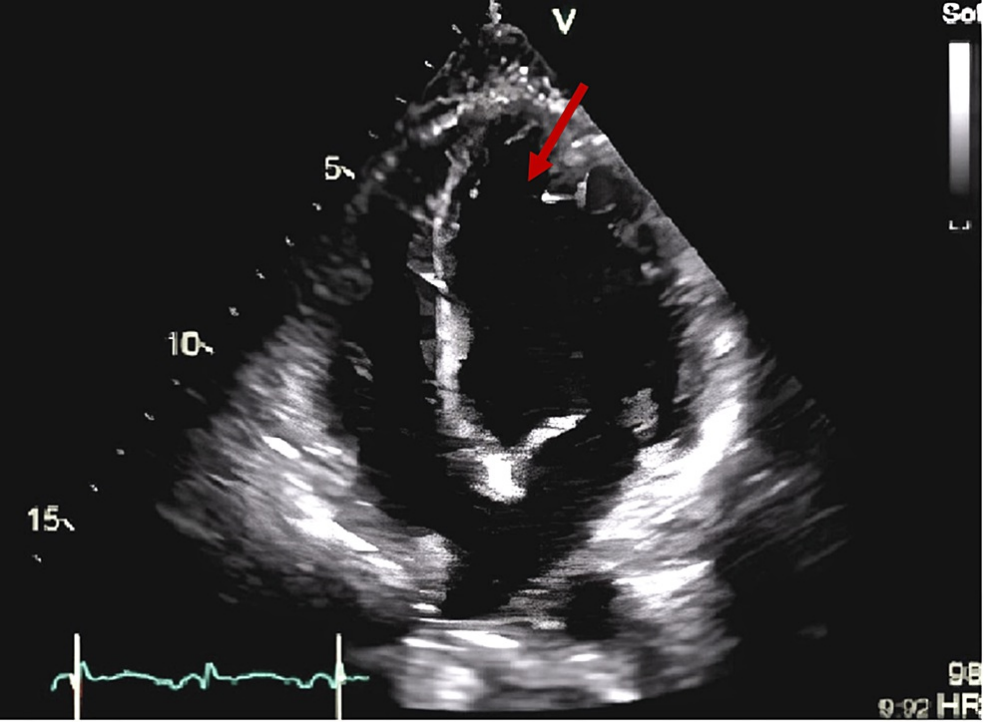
Transthoracic echocardiography Moderately to severely compromised systolic left ventricular function (ejection fraction of 32%), with mid-distal akinesia (arrow) and basal hypercontractility.
